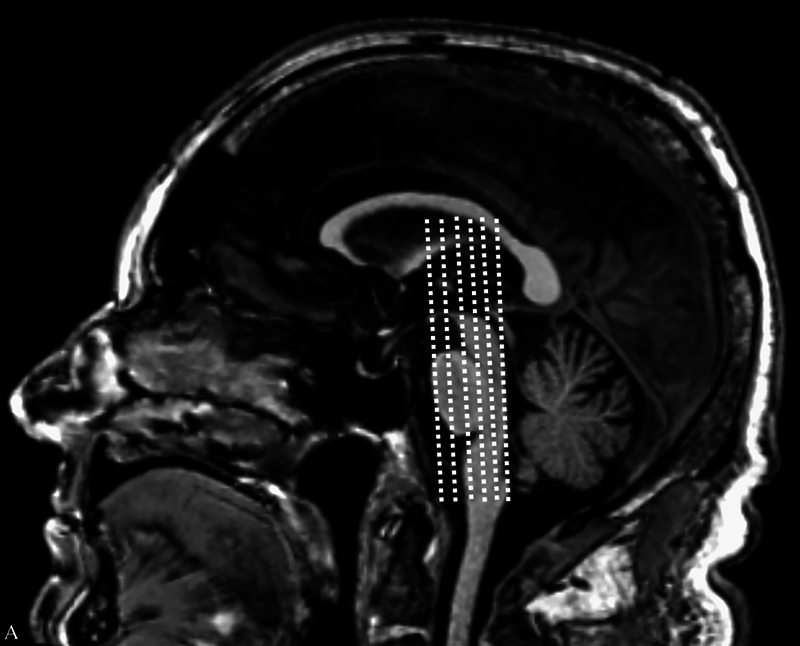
Evaluation of T1-weighted images in multiplayer reconstruction, from the level of the uncus-amygdala complex anteriorly to the dorsal hippocampus posteriorly, at the level of the mammillary bodies.
